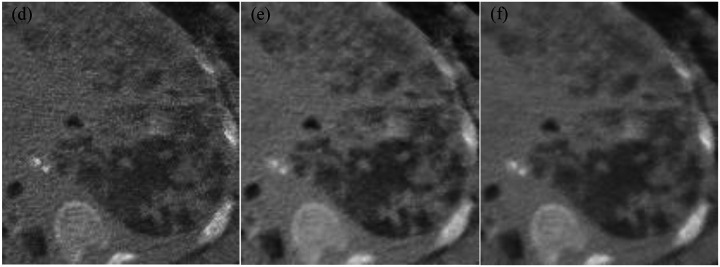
Comparison of CT image reconstructions from clinical raw data of 492 views. (a) The image reconstructed using SART, (b) the image reconstructed by the score function-based method [16], and (c) the image reconstructed by the ISM-based method, and (d-f) enlarged image for red rectangle in images (a-c), respectively.
